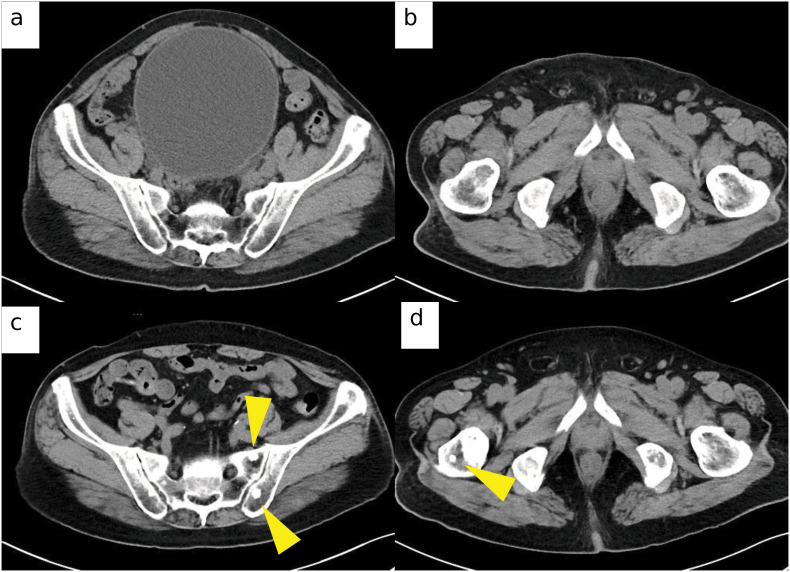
CT images demonstrating disease course prior to olaparib initiation. (a) Axial CT image at initial diagnosis demonstrating osteoblastic metastasis in the pelvic bone. (b) Axial CT image at initial diagnosis showing osteoblastic metastasis in the femur. (c) Axial CT image obtained at prostate-specific antigen (PSA) progression during enzalutamide therapy, demonstrating increased osteoblastic lesions consistent with radiologic progression. (d) Axial CT image at PSA progression during enzalutamide therapy confirming further progression of sclerotic bone metastases. All images are axial CT scans acquired with a 5-mm slice thickness.
